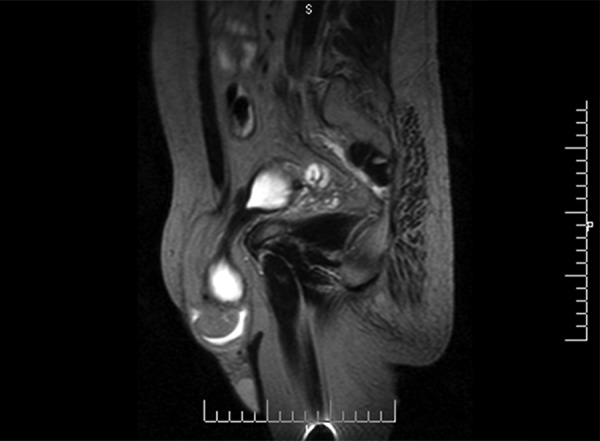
Magnetic resonance imaging (parasagittal section) showing left inguinoscrotal bladder herniation
